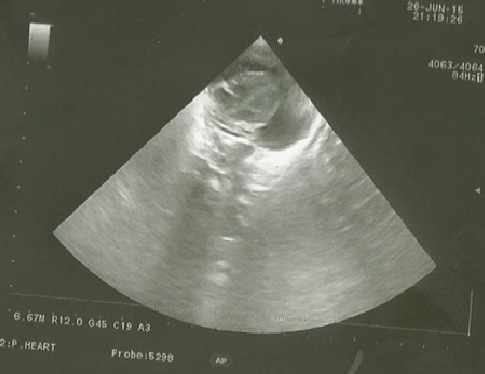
Four chamber echocardiography view showing a significant pericardial effusion
